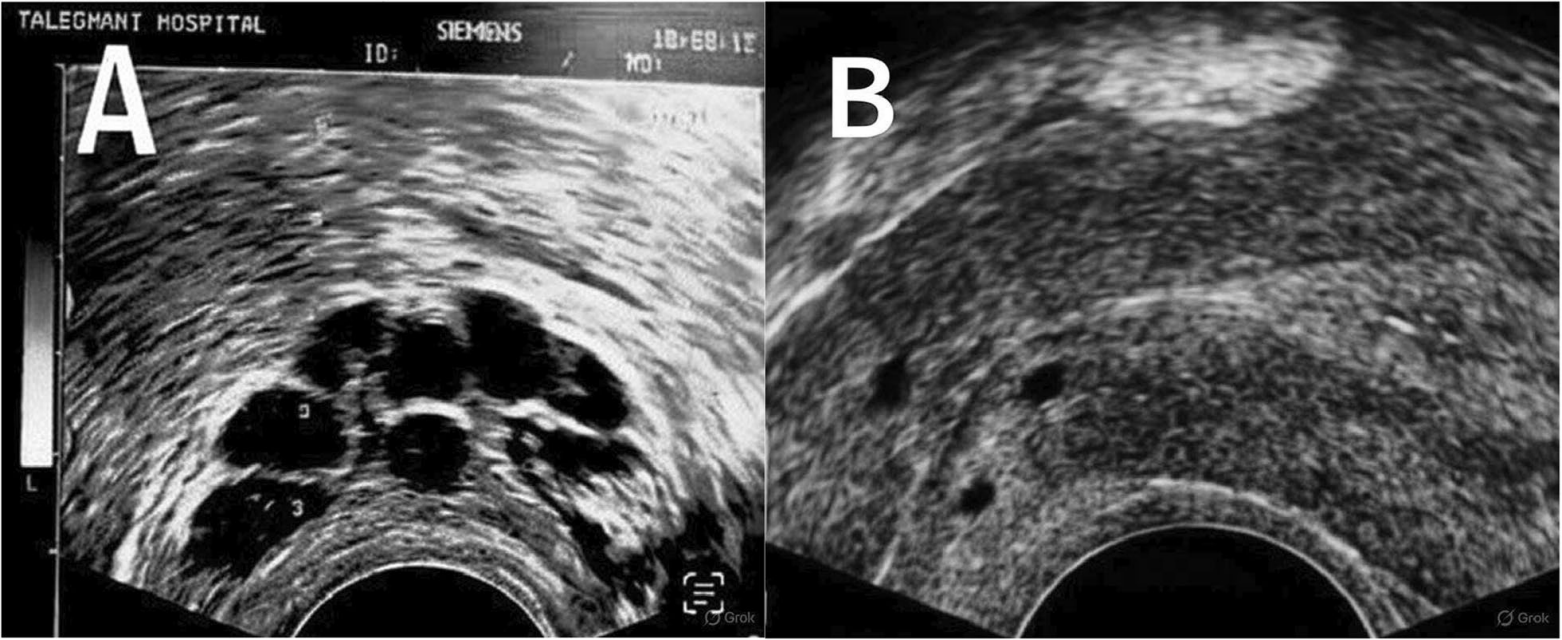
Transvaginal ultrasound images showing the cervical cysts (A) before and (B) after aspiration
